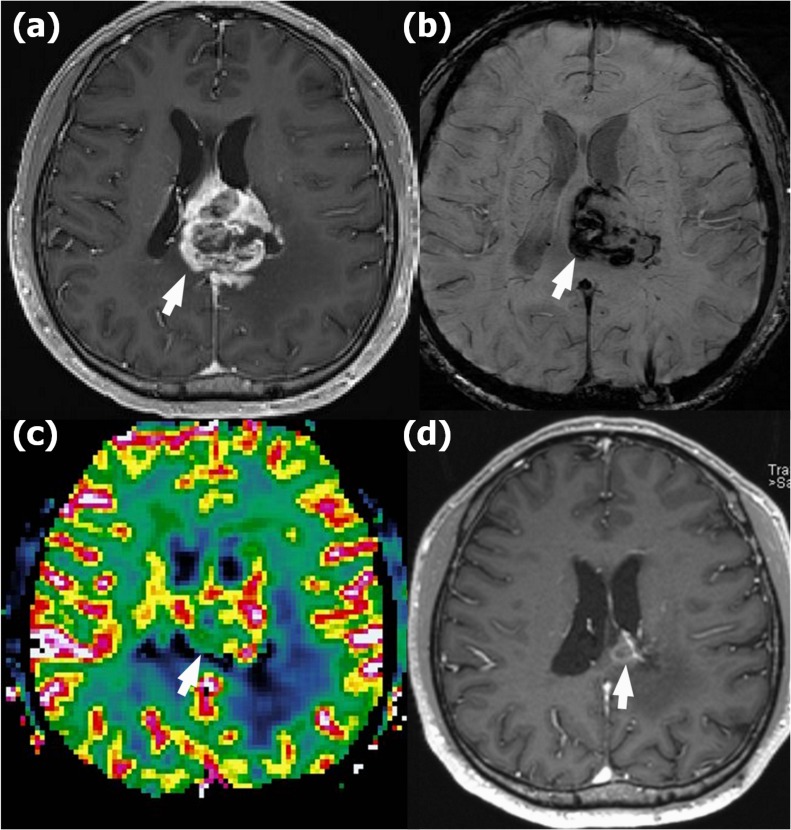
Radionecrosis in a 41-year-old man with glioblastoma in the left mid corpus callosum who underwent gross total resection and concomitant chemoradiotherapy (CCRT) a. Contrast-enhanced T1-weighted (CET1) magnetic resonance (MR) image obtained 18 months after CCRT completion shows newly appearing enhancing lesions in the left mid corpus callosum (arrow). b. Susceptibility-weighted imaging demonstrates significant dark areas in the corresponding enhancing lesions (arrow); the proportion of dark signal intensity (proSWMRI) was 40.5 %. c. The normalized relative cerebral blood volume map (nCBV) from dynamic susceptibility contrast perfusion-weighted imaging shows increased blood flow in the corresponding enhancing area (arrow) (calculated 90^th^ percentile points in the cumulative nCBV histogram (nCBV90) = 2.84). d. Follow-up CET1 MR image obtained 36 months after the first appearance of the enhancing lesion shows an interval decrease in the extent of the enhancing lesion (arrow), suggesting radionecrosis.
